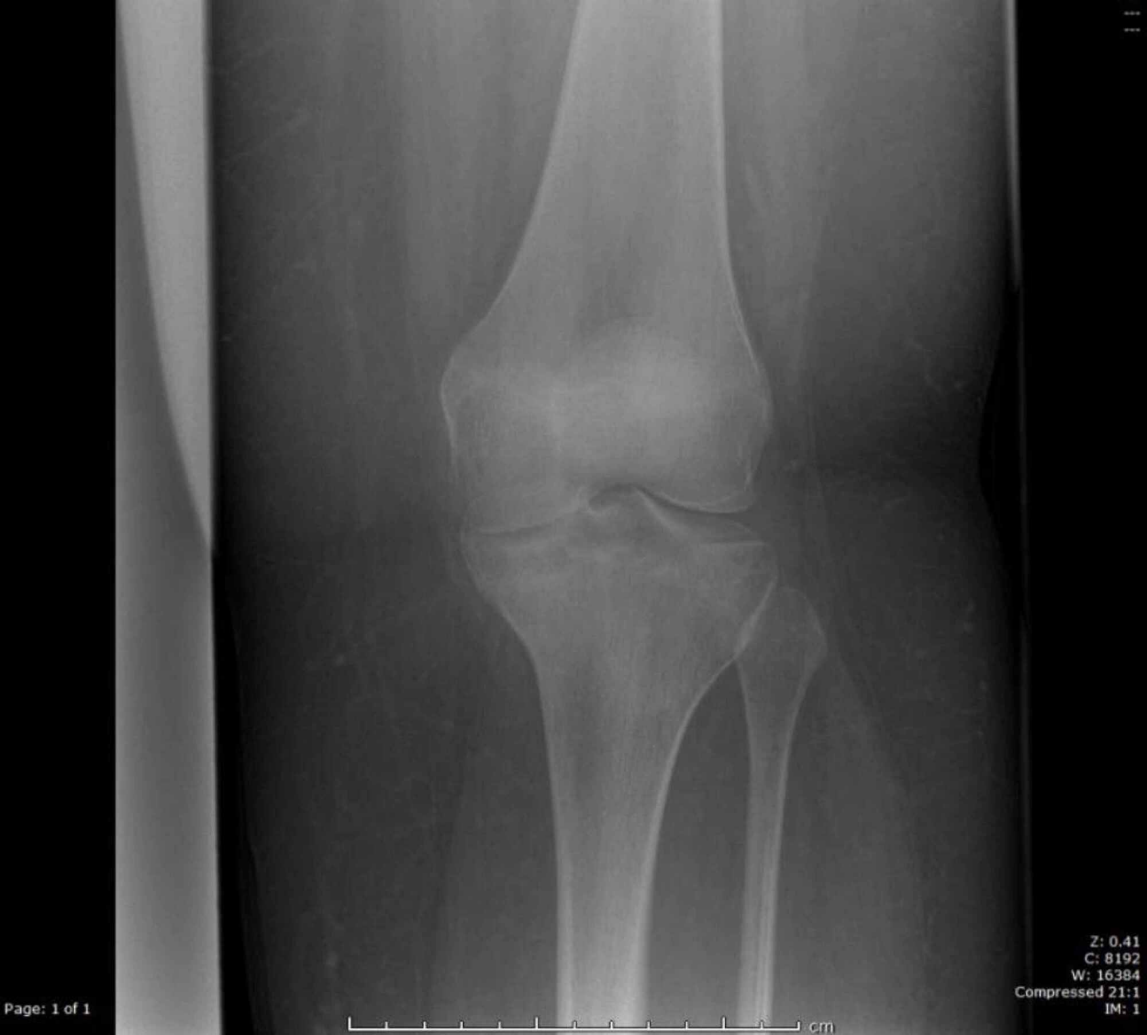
Radiograph of left knee at 12 weeks post injury (anterior posterior view)
